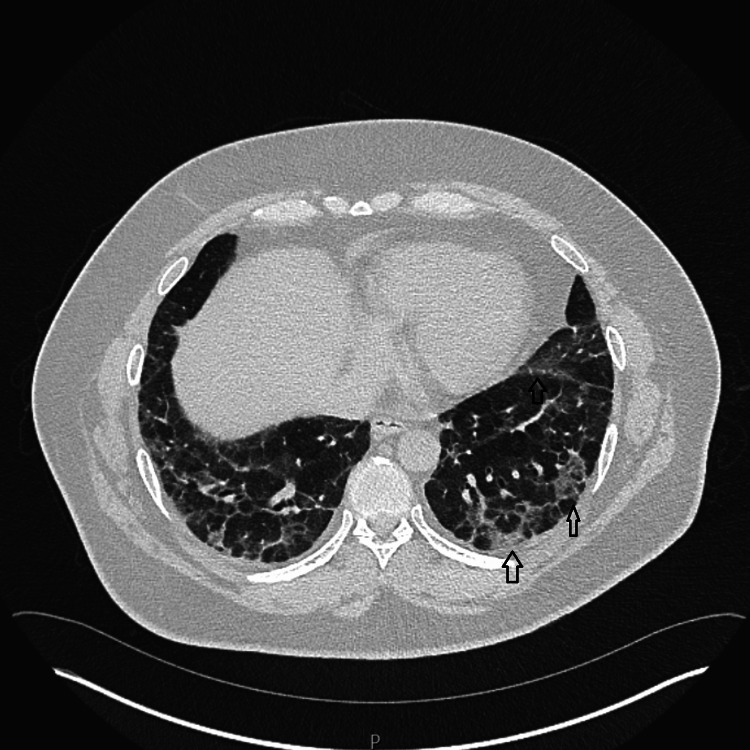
Follow-up HRCT chest: Improvement of ground-glass opacities, consolidations, and traction bronchiectasis. HRCT: high-resolution CT
